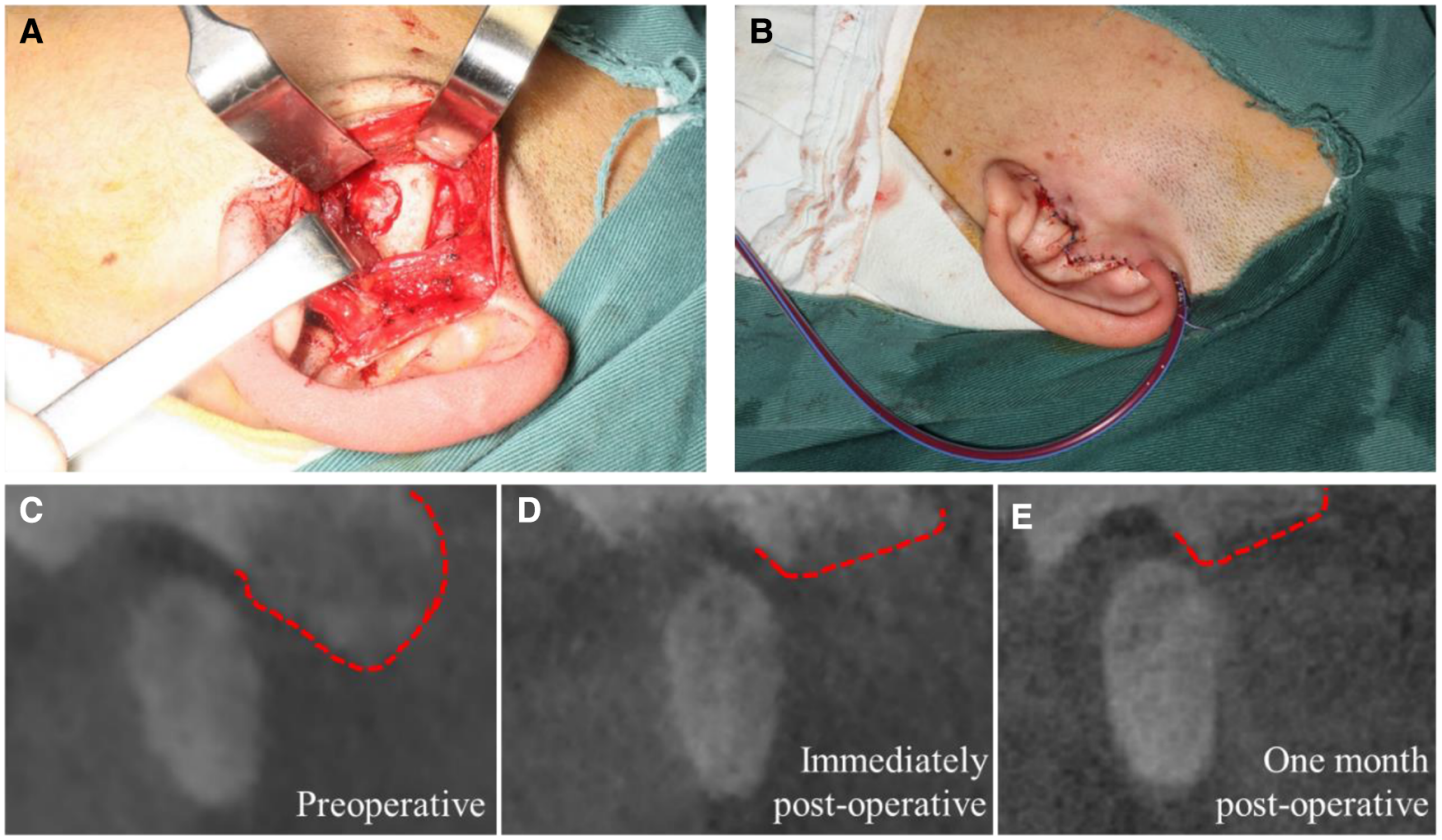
Surgical intervention and radiological change in CBCT images: (A) lesion resection at the left articular tubercle; (B) stitches of the incision and high vacuum drainage device placement; (C–E) CBCT images of the lesion area preoperatively, immediately postoperatively and one month postoperatively, respectively. (Red dotted lines show the border the articular tubercle region).
